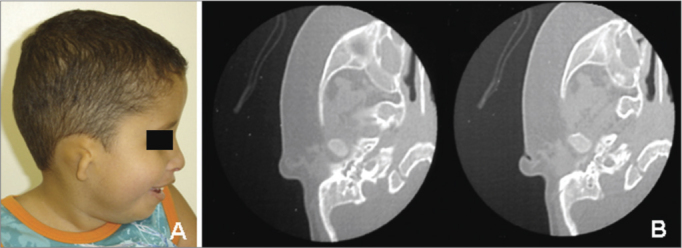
OAVS patient from the study sample (number 12 in Table 1) - the computed tomography of the mastoid showing right ear anomalies (opacified middle ear and malformed ossicular chain).
